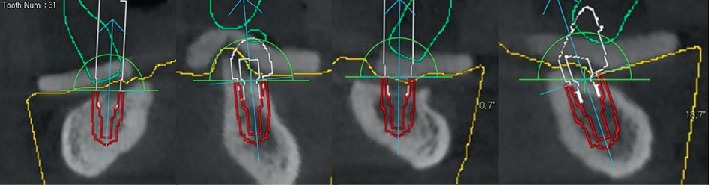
The CBCT scan. Coronal mandibular view. Four implants were placed in the 3.2, 3.4, 4.1, and 4.3 teeth regions. Distal implants are tilted at 29 degrees.
